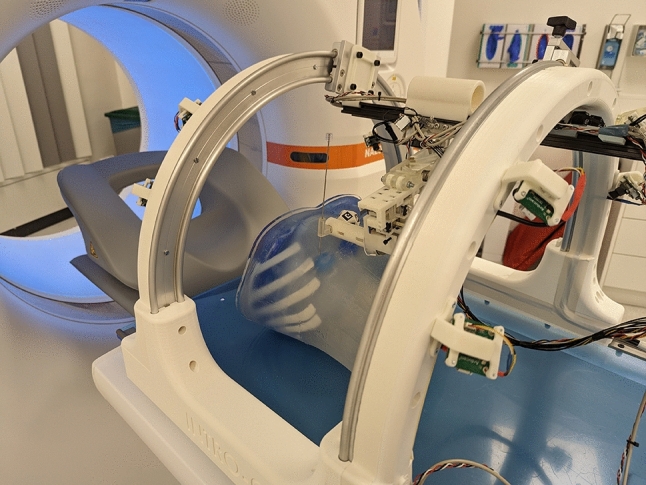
Robotic device on the CT scanner table
